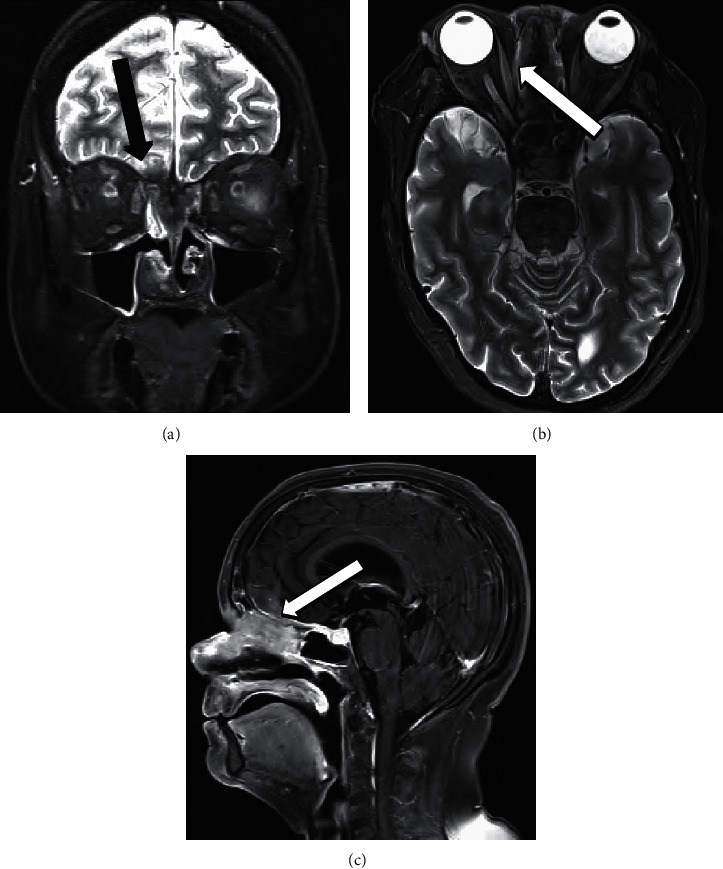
Triplanar MRI coronal STIR (a), axial STIR (b), and sagittal (c) T1-flair with fat saturation postcontrast showing midline anterior skull base mass with extension through skull base and right lamina papyracea (arrows) and saddle nose deformity (star).
